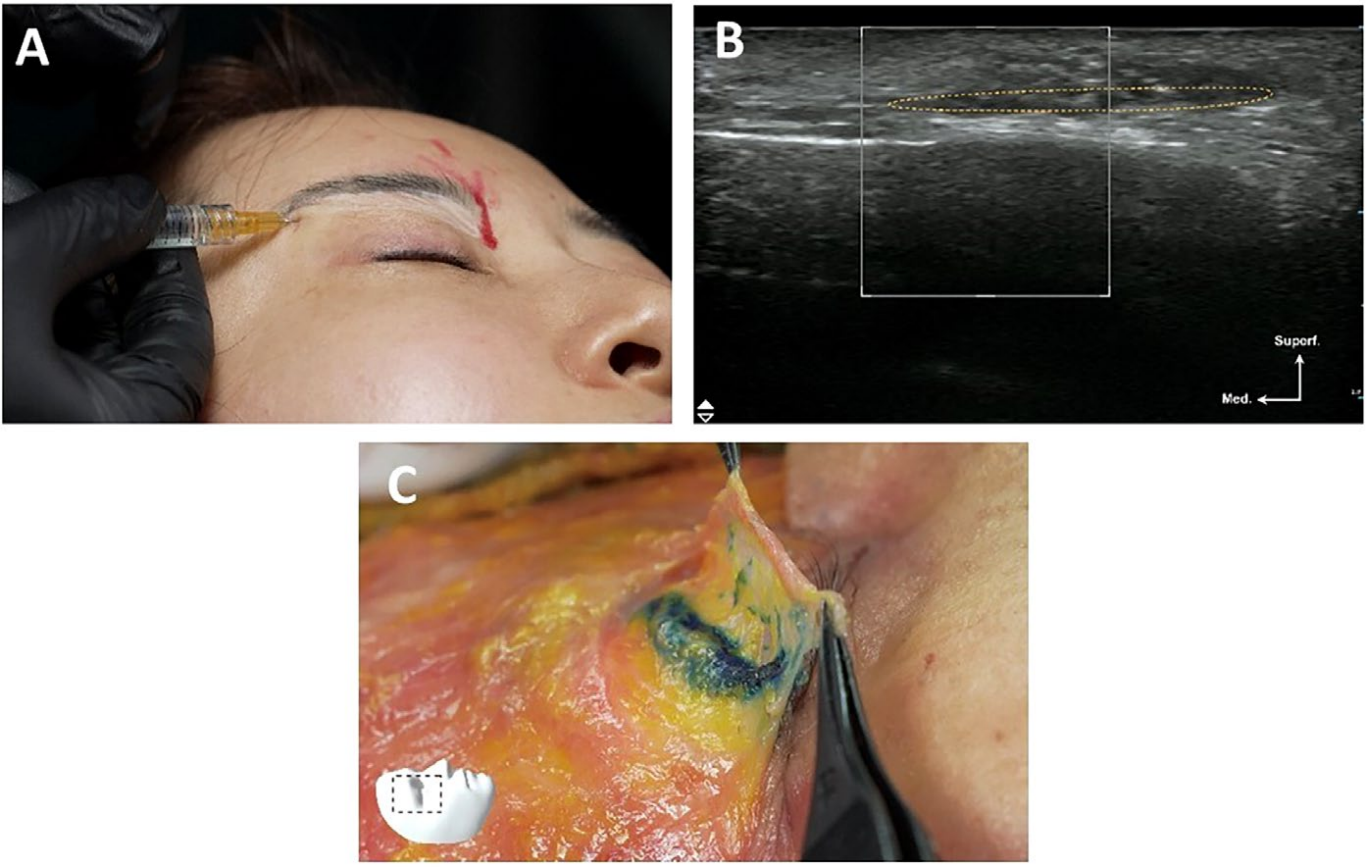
A demonstration of deep‐plane injection of filler in the upper eyelid (41‐year‐old Korean female). (A) Clinical injection. (B) Clinical ultrasound (C) Cadaver dissection (72‐year‐old Korean male).
